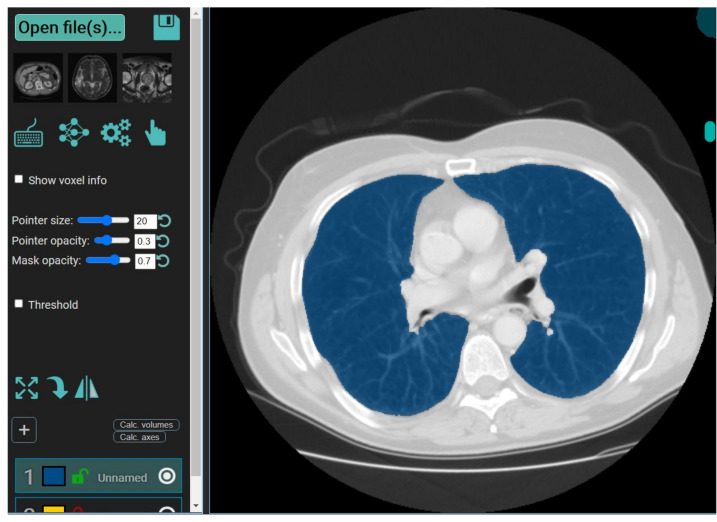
Segmentation of the lung in CT slice using the MedSeg tool.
